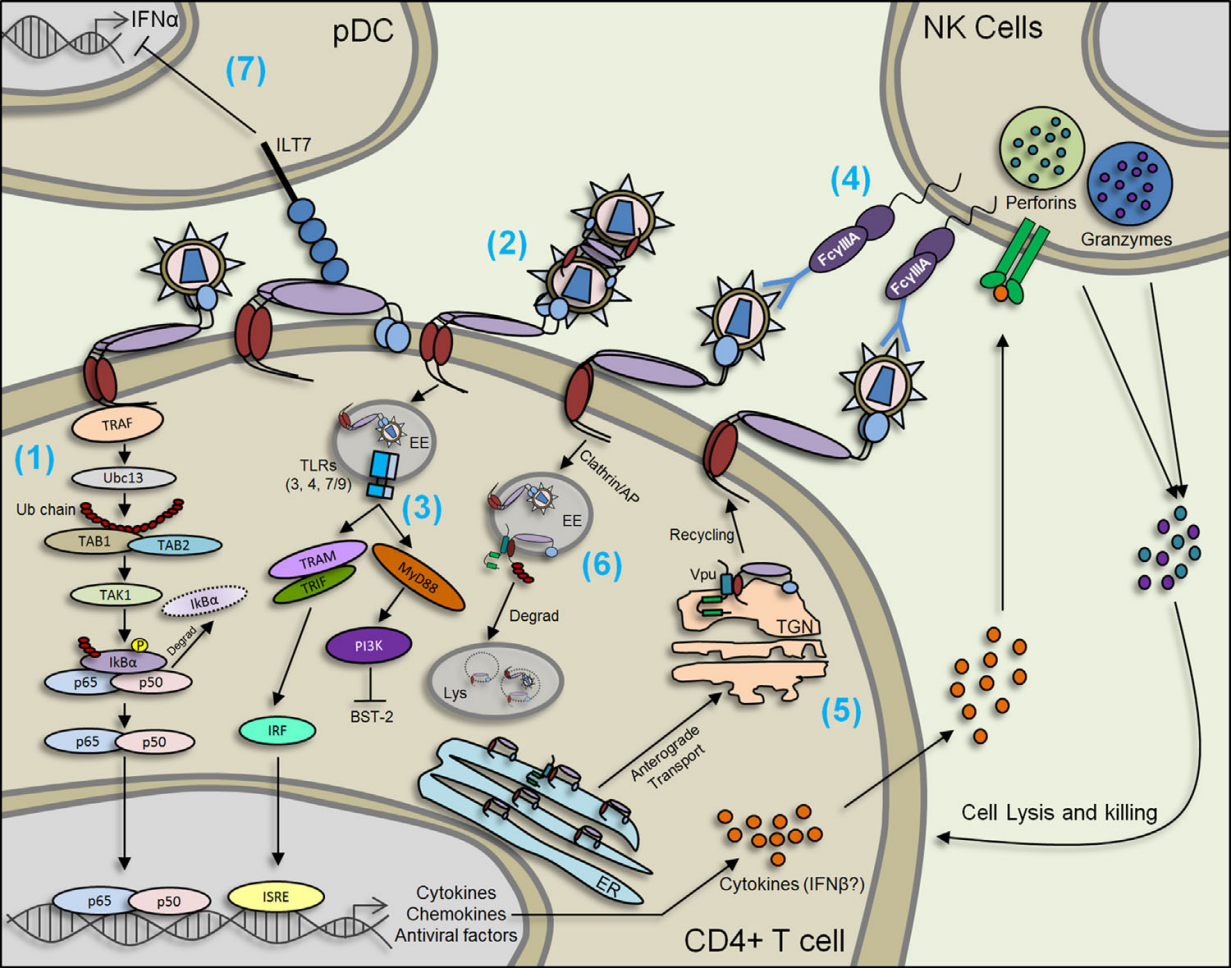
BST‐2 in viral infection: BST‐2 tethers viruses to the cell membrane and to each other (1) and (2). Tethered viruses may be internalized through clathrin coated pits mediated by the YXY motif on BST‐2's cytoplasmic tail (CT). Virus tethering by BST‐2 also induces the activation of NF‐κB through BST‐2's YXY motif which interacts with TRAF and requires Ubc13, Tab1, Tab2, and TAK1 (1). NF‐κB activation induces the expression of cytokines, such as CXCL10 and Il‐6 and may induce the expression of BST‐2 since the BST‐2 promoter contains elements for NF‐κB binding (1). In the early endosome (EE), TLRs recognize different viral factors and induce the expression of cytokines, chemokines and several antiviral factors that contain IFN‐sensitive response elements (ISRE) on their promoter (3). Signaling through TLRs regulates BST‐2 in a positive and/or a negative manner (3). BST‐2 tethering is also required for the induction of antibody‐dependent cellular cytotoxicity (ADCC) which in turn induces the degranulation of effector cells, such as natural killer (NK) cells, through the FcγIIIA receptors (4). Viruses have evolved mechanisms to antagonize BST‐2. The best studied BST‐2 antagonist is Vpu which interacts with BST‐2 at the endoplasmic reticulum (ER), trans‐Golgi network (TGN) and early endosomes. Vpu sequesters BST‐2 at all of these sites. Vpu can also prevent anterograde transport of BST‐2 from the ER to the TGN, and prevent BST‐2 recycling from TGN to the plasma membrane (5). Vpu also promotes BST‐2 degradation (Degrad) through the lysosomal pathway (Lys = lysosome) (6). BST‐2 is a ligand for ILT7 (immunoglobulin‐like transcript 7) at least in plasmacytoid dendritic (pDC) cells. Engagement of ILT7 by BST‐2 represses IFNα expression which in turn can induce BST‐2 levels (7).
